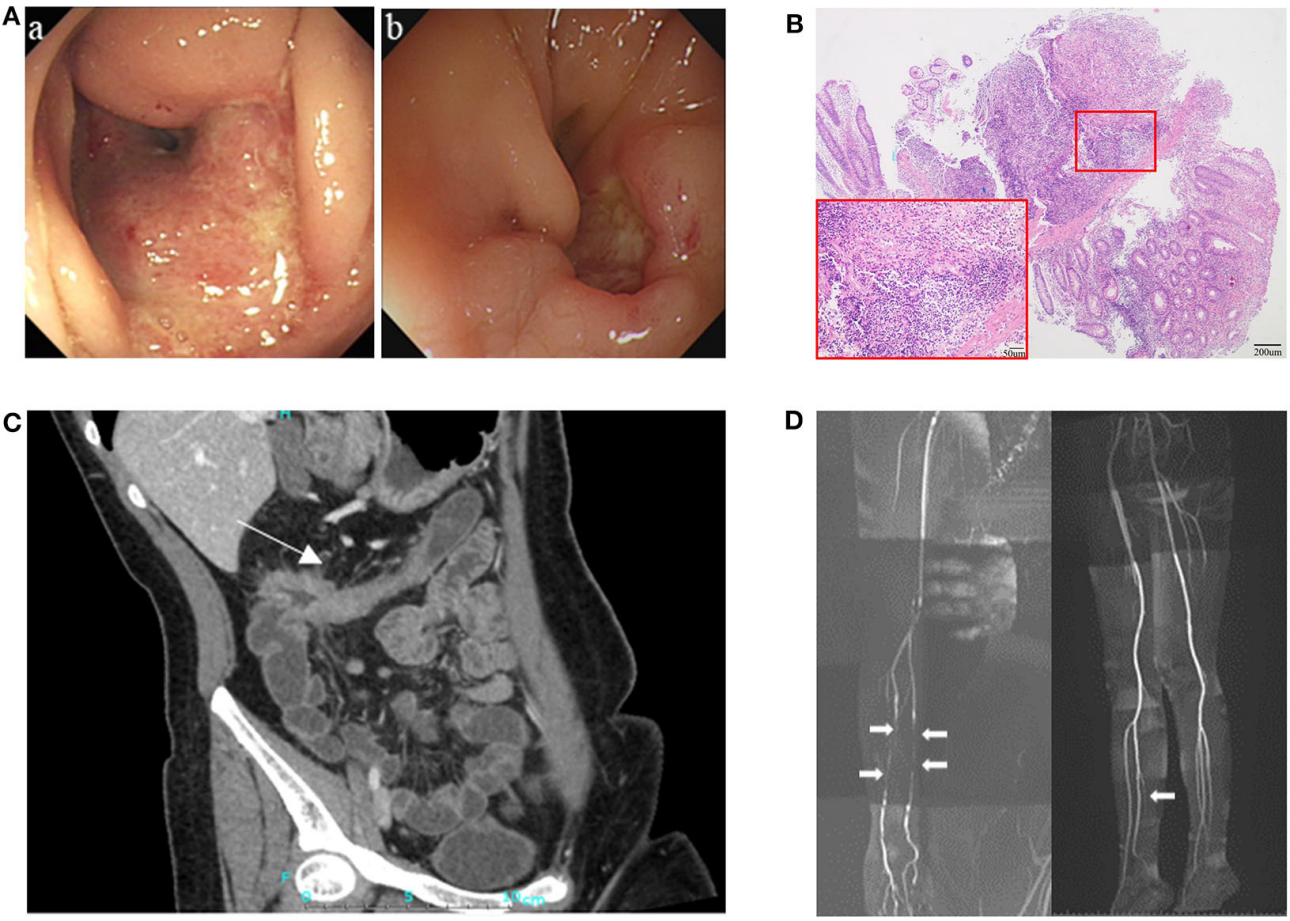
Clinical presentation of the patient. (A) Endoscopy showed ulcers and stricture in the ascending colon in August 2010 (a), and relapsing ulceration of intestinal anastomosis and stricture in transverse colon 8 years after right hemicolectomy (b). (B) Pathological examination showed intestinal transmural inflammation with an increased number of inflammatory cells infiltration, loss of crypt and hyperplasia of fibrous tissue. (C) CT enterographic (CTE) image demonstrated thickening of the wall and stricture in the colon-hepatic curvature and the proximal transverse colon (arrow). (D) Magnetic resonance angiography (MRA) showed multiple arteritis stenosis in upper and lower limbs (white arrows).
